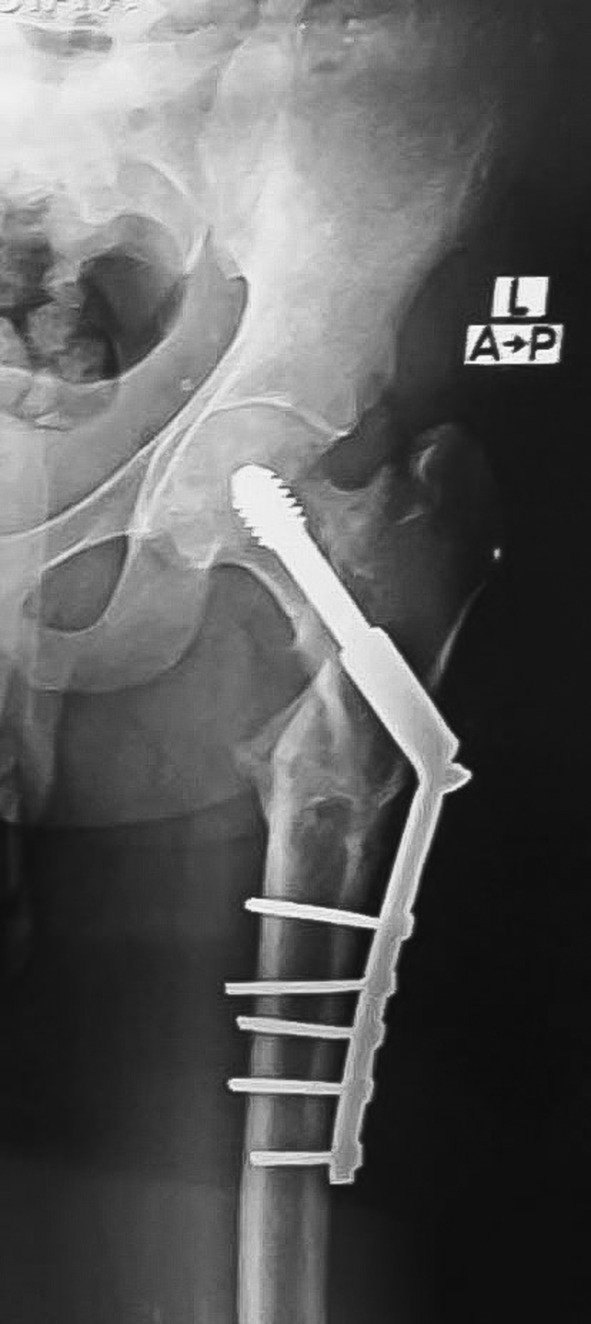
Antero‐posterior radiograph of the same patient after 2 years, post‐operatively, shows a well‐healed fracture and osteotomy sites.
